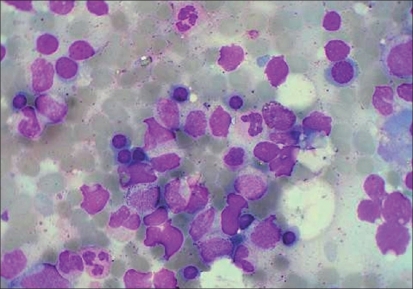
CT-guided biopsy from lung lesion showing hyperplastic erythroid bone marrow
